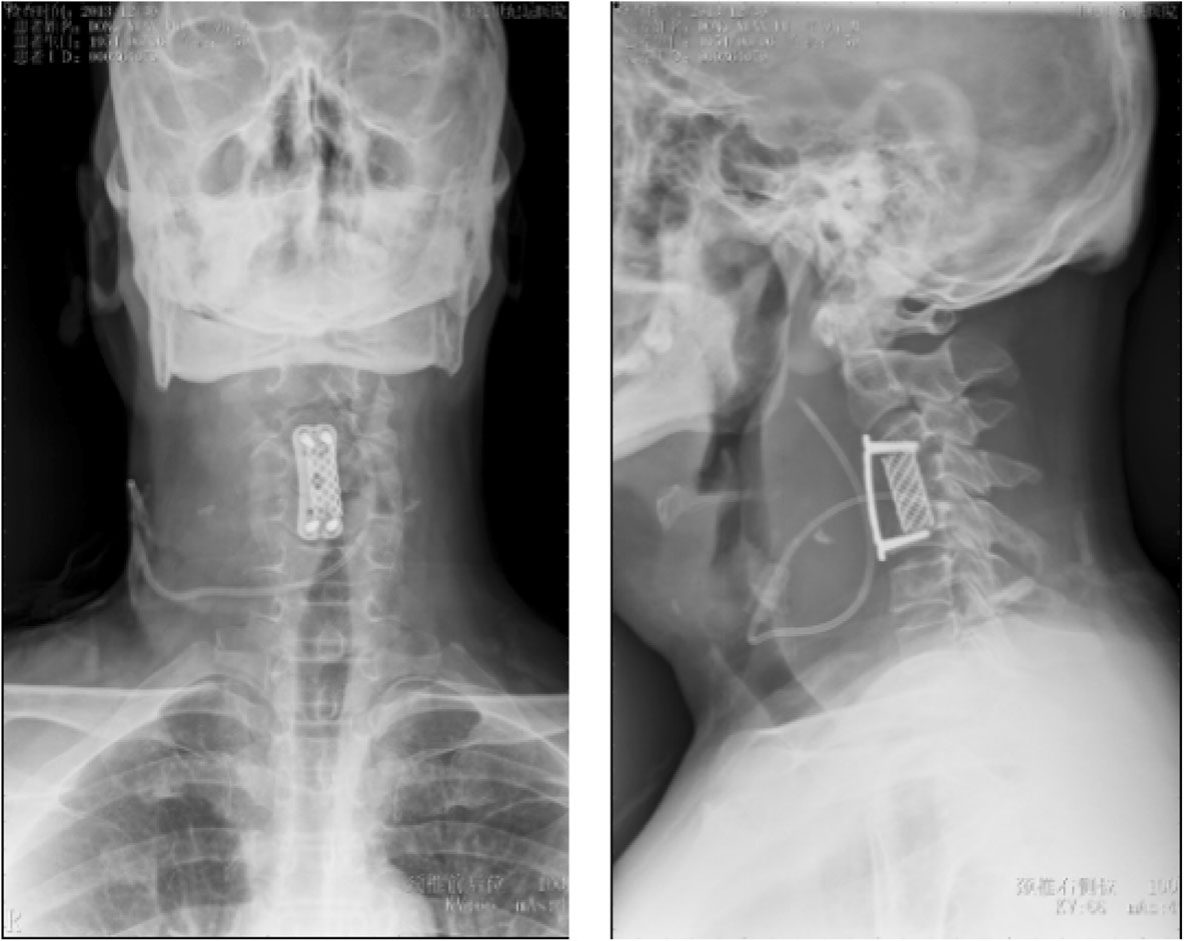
Plain radiograph of neck and implant on admission
